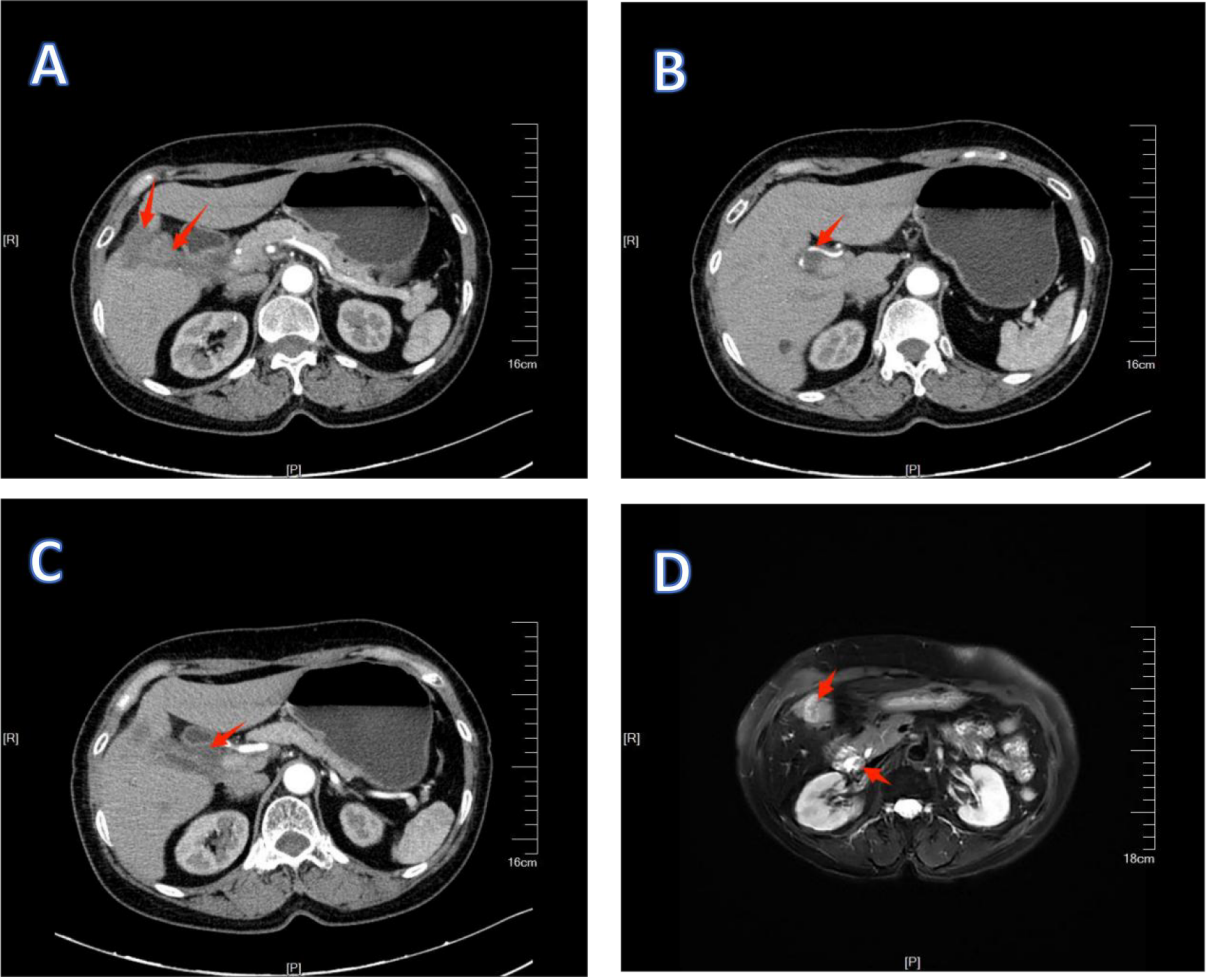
1Preoperative examination of patient 2. (A–C) are CT images. (D) is an MRI image. (A) shows the reduction of the primary lesion and the metastatic lesion. (B) shows an improvement in the condition where the lymph nodes surround the hepatic artery. (C) shows a reduction and shrinkage of lymph node metastasis around the hepatic hilum.
